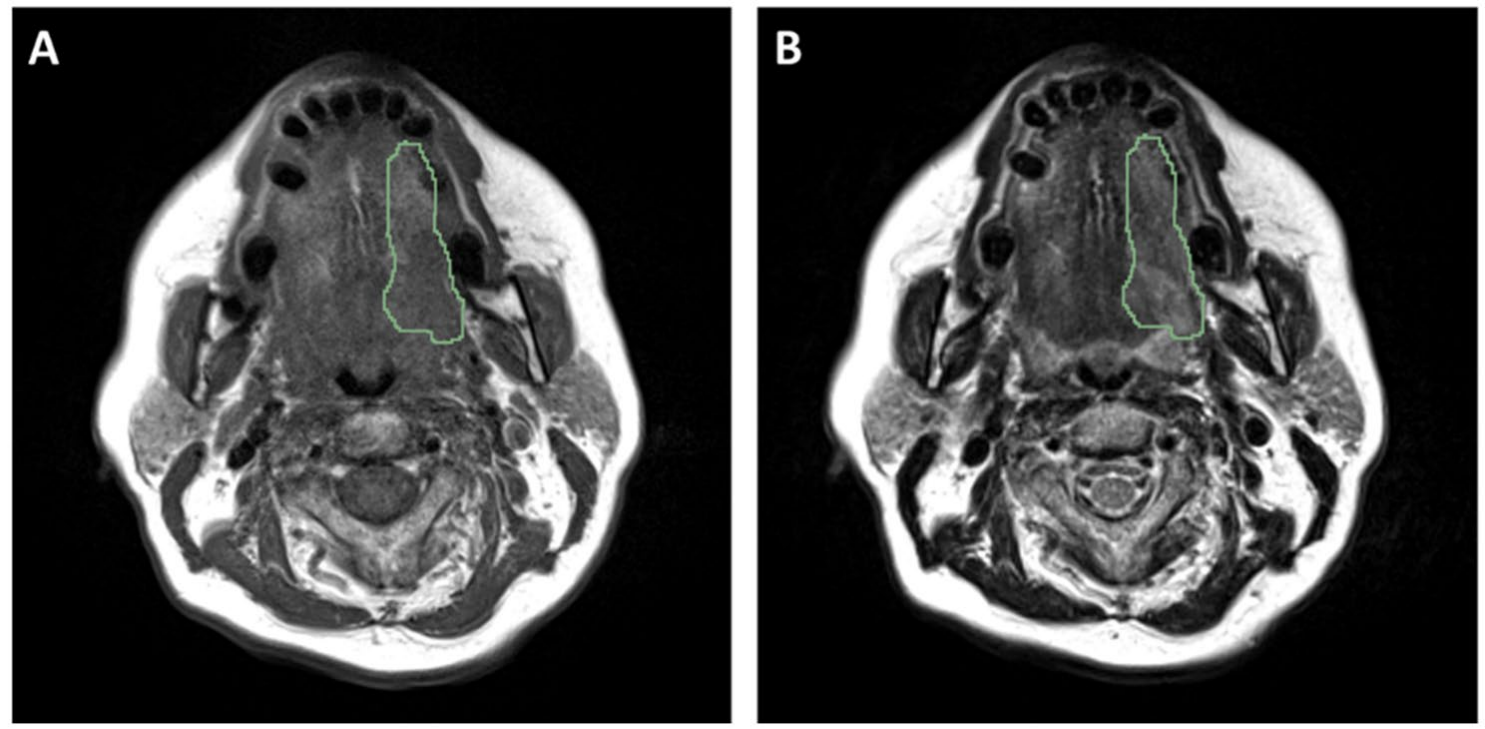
Type of magnetic resonance images acquired for the study: (A) T1-weighted image; (B) T2-weighted image. The segmented region of interest is also displayed
